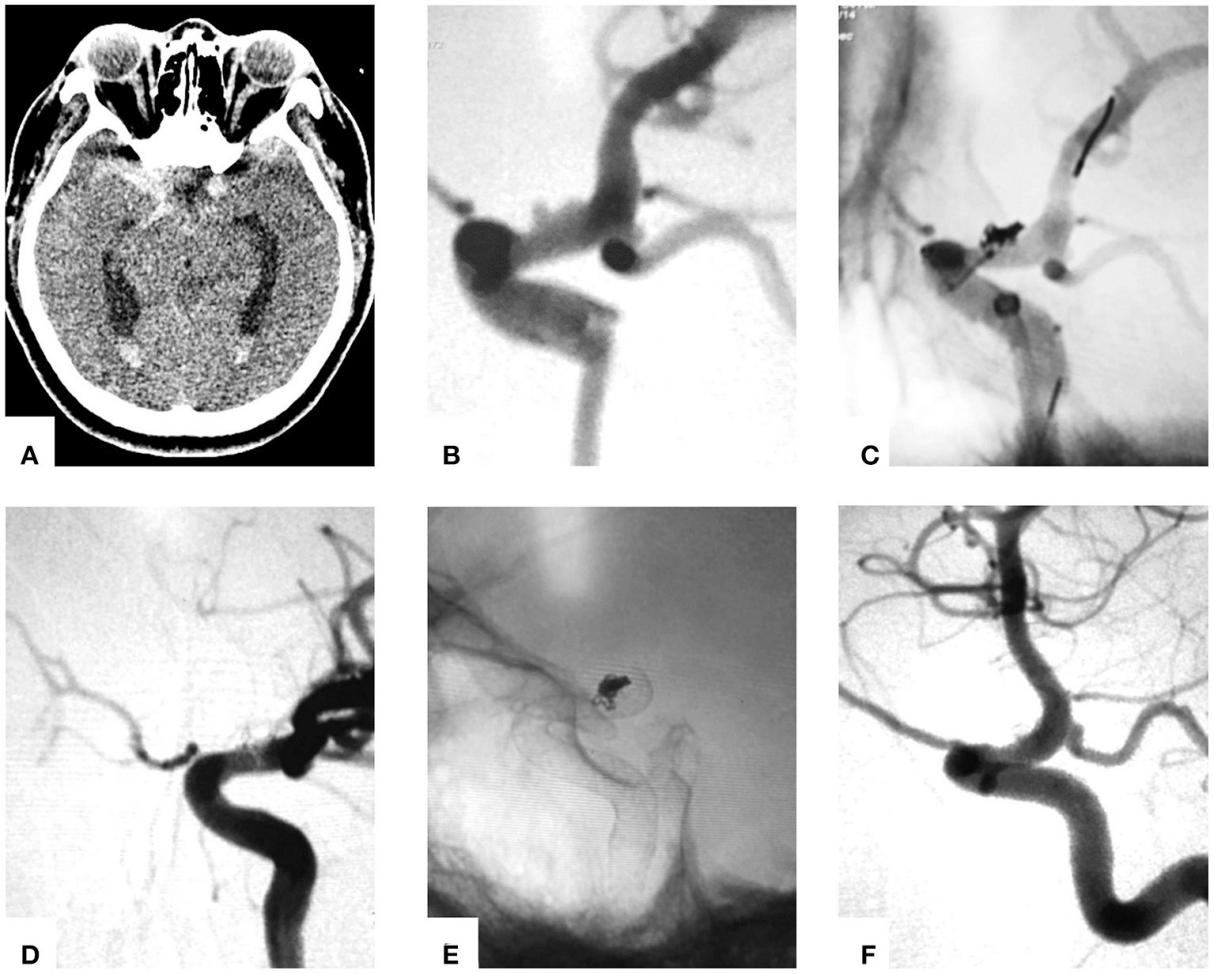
Images of the typical cases. (A) CT scan showed symmetric suprasellar cistern SAH and hemorrhage in lateral ventricles. (B) Cerebral angiograms obtained immediately pre-embolizaton. (C) PED semi-deployed coiling. (D) and (E) Post-treatment DSA. (F) DSA follow-up 6 months after treatment.
